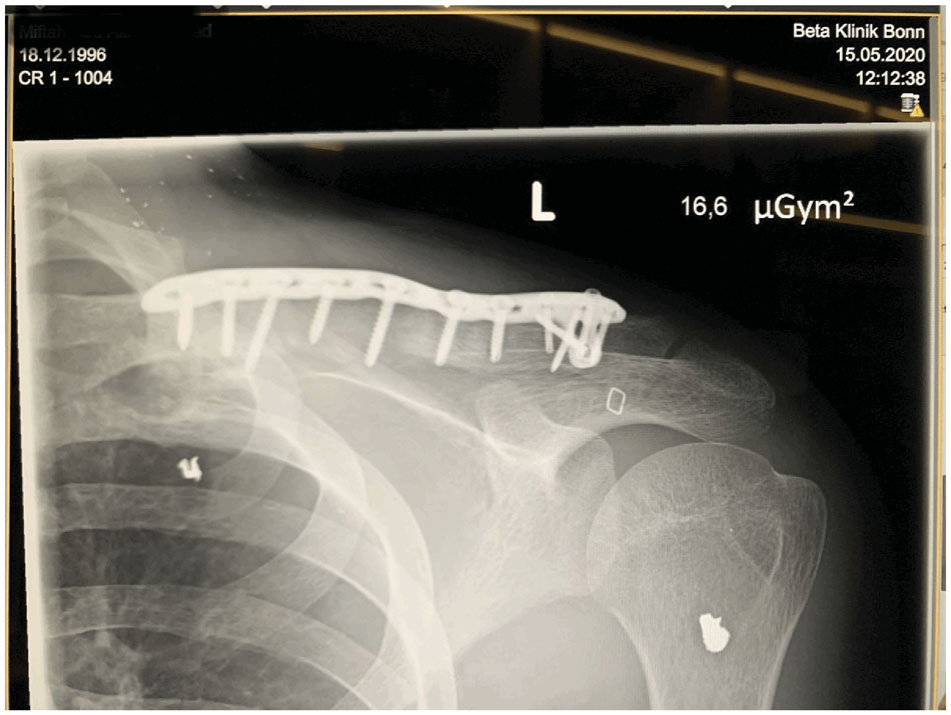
X-ray imaging 8 weeks after surgery shows good embedding of the bone graft and regular plate and screw positions.
